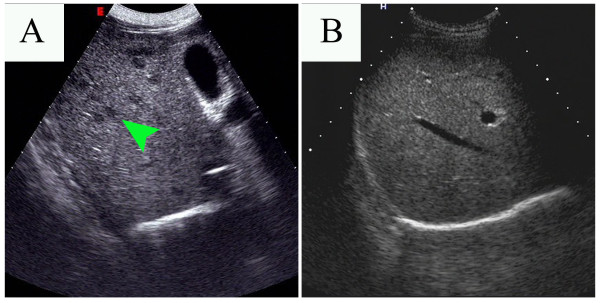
Abdominal ultrasound results before and after the first therapy. (A) Before therapy, multiple small hypoechoic nodular lesions were detected (arrow) in the patient’s liver. (B) The result of the ultrasound after the first anthelmintic therapy was normal.
